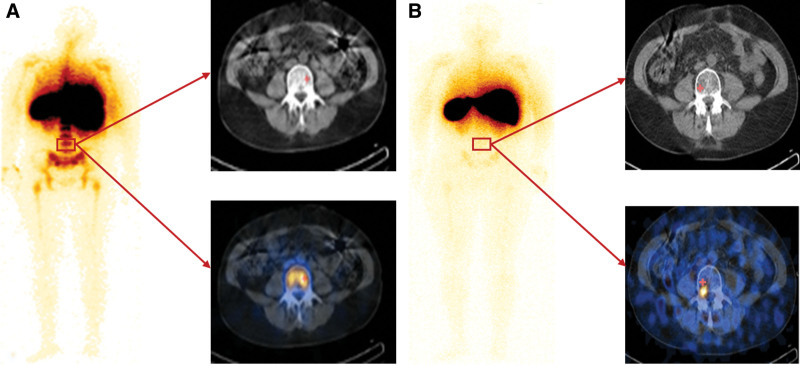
A 46-year-old female patient with moderately differentiated squamous cell carcinoma of the cervix following surgery (FIGO stage IB2). BM scintigraphy showed uniform high uptake in the lumbar region before CRT treatment (A, a qualitative grading of grade 3) and little uptake after treatment (B, a qualitative grading of grade 0). BM = bone marrow, CRT = chemoradiotherapy.
